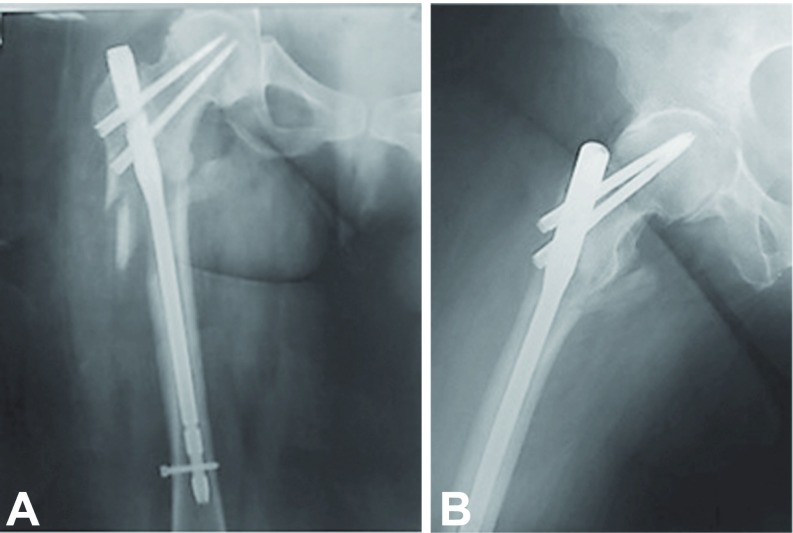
(A) Anteroposterior radiograph of the right hip of an 80-year-old woman with an unstable pertrochanteric fracture (reverse oblique, AO/OTA 31A3.3) of the left hip treated with long intramedullary hip nail. (B) Lateral radiograph of the right hip at 3-month follow-up shows fracture healing; the patient was weight-bearing with a walker.
